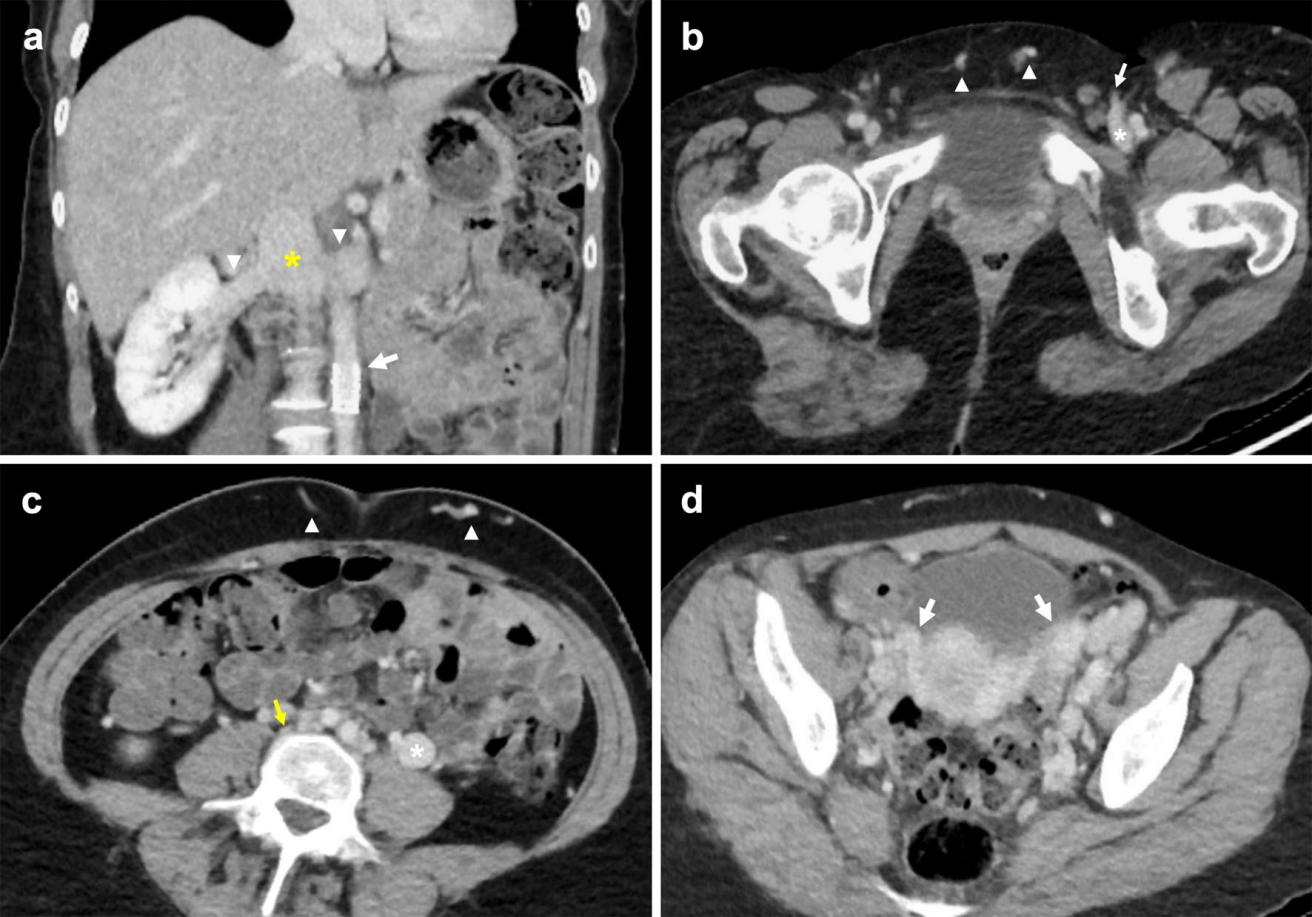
Absent infrarenal IVC with multiple collateral pathways. Contrast-enhanced delayed venous phase on a CT abdomen and pelvis demonstrates prominent superficial abdominal, epigastric and gonadal veins draining the lower extremities. a Coronal CT image shows paired renal veins (arrowheads) draining into the caudal end of the IVC (asterisk). An aortic stent (arrow) is visualised inferior to the left renal vein. b Axial CT shows prominent external pudendal veins (arrowheads), and the left femoral vein (asterisk) receiving a distended superficial inferior epigastric vein (arrow). c Axial CT image with a distended left gonadal vein (asterisk). Small calibre unenhanced infrarenal IVC (arrow) is suggestive of chronic occlusion. Enhanced right inferior epigastric and abdominal wall veins are also visualised (arrowhead). d Axial CT demonstrates bilateral enlarged pelvic veins (arrows)
